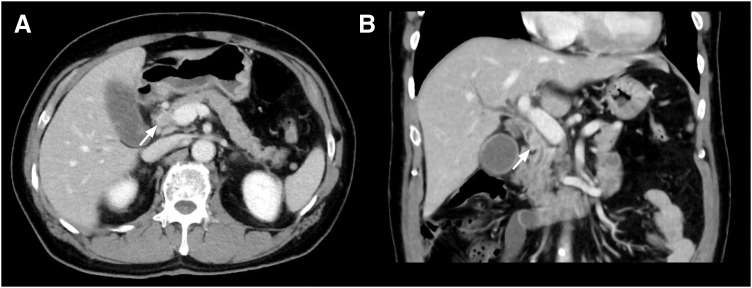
Findings of contrast-enhanced CT. Contrast-enhanced CT revealed a well-enhanced tumor in the middle bile duct (arrow) in the axial image (A) and coronal image (B).
